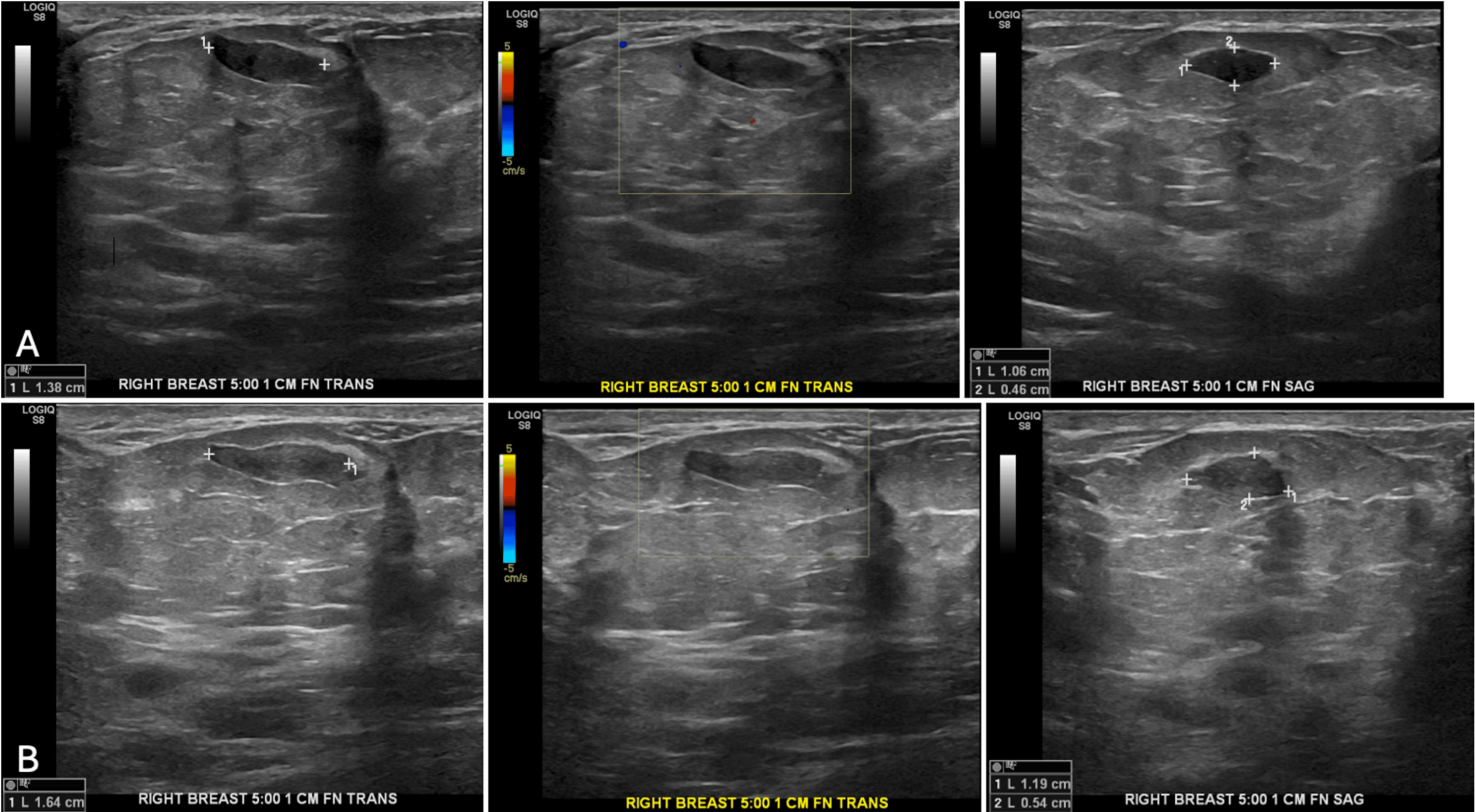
Ultrasonography of the breast mass in the right breast at 5 o'clock A. Two months after the initial mammogram, a follow-up ultrasound demonstrated a 1.4 x 0.5 x 1.1 cm oval mass with circumscribed margins within a hamartoma. B. Nine months after the initial mammography, the same oval mass was grossly stable and measured 1.6 cm x 0.5 cm x 1.2 cm.
